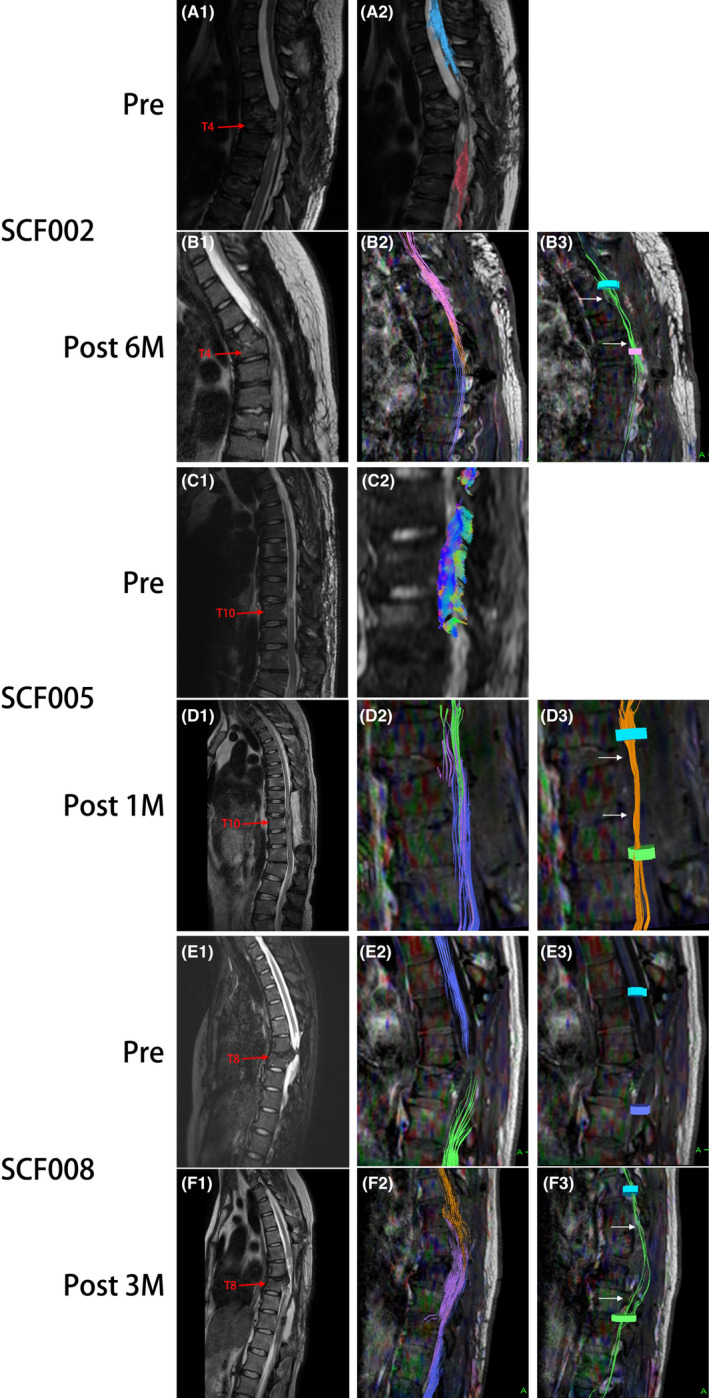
Representative neuroimaging images of participants SCF002, SCF005, and SCF008 treated with vSCT. Preoperative T2‐weighted MRIs images show significant spinal cord injury (A1, C1, E1). DTI images show complete disruption of the spinal cord fibers (A2, C2, E2, E3). Postoperative T2‐weighted MRI images show the vascularized transplanted spinal cord in the original SCI area (B1, D1, F1). The three colored nerve fibers shown by DTI images represent nerve tracing and imaging of the distal and proximal spinal cord and the vascularized transplanted spinal cord (B2, D3, F3). DTI images show the reconnection of some nerve fibers to restore the nerve continuity of the spinal cord (B3, D3, F3). Pre, Preoperatively; Post 1 M, 1 month postoperatively; Post 2 M, 2 months postoperatively; 6 M: 6 months postoperatively
